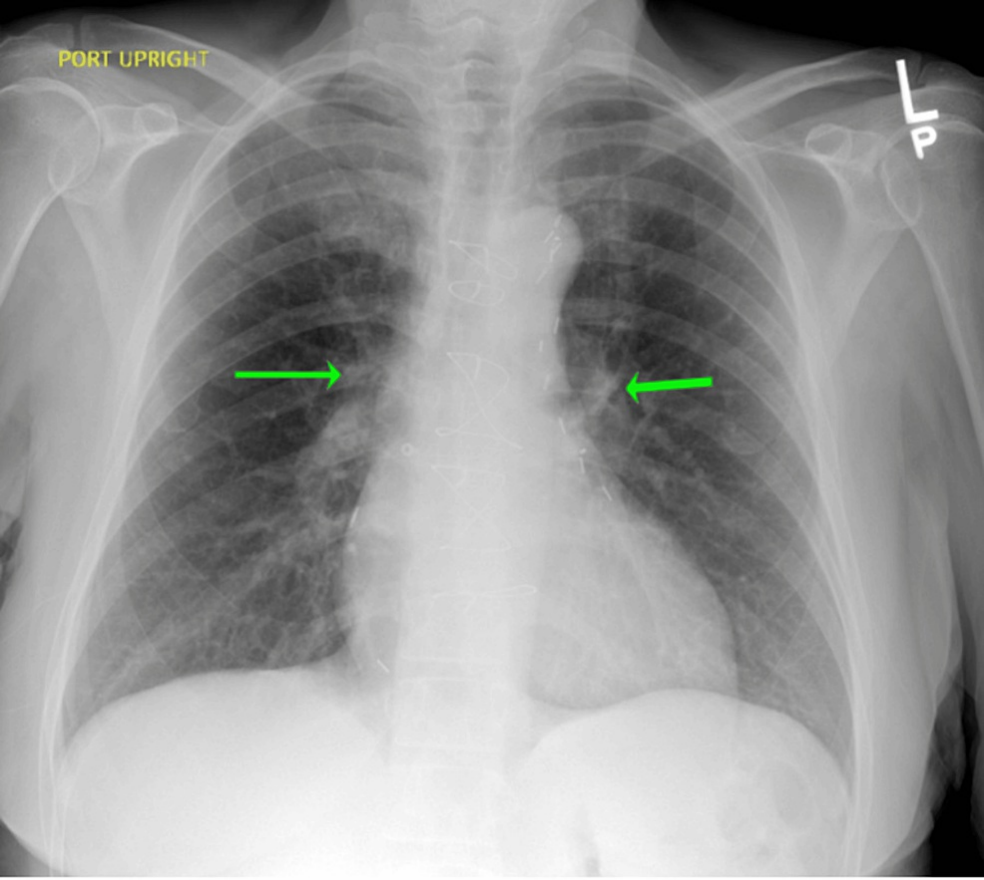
Chest radiograph anterior-posterior view showing no evidence of pulmonary infiltrates, pleural effusions, or pneumothorax. The heart size is in the upper limits of normal with evidence of previous cardiac surgery. There is prominence of central pulmonary vessels
